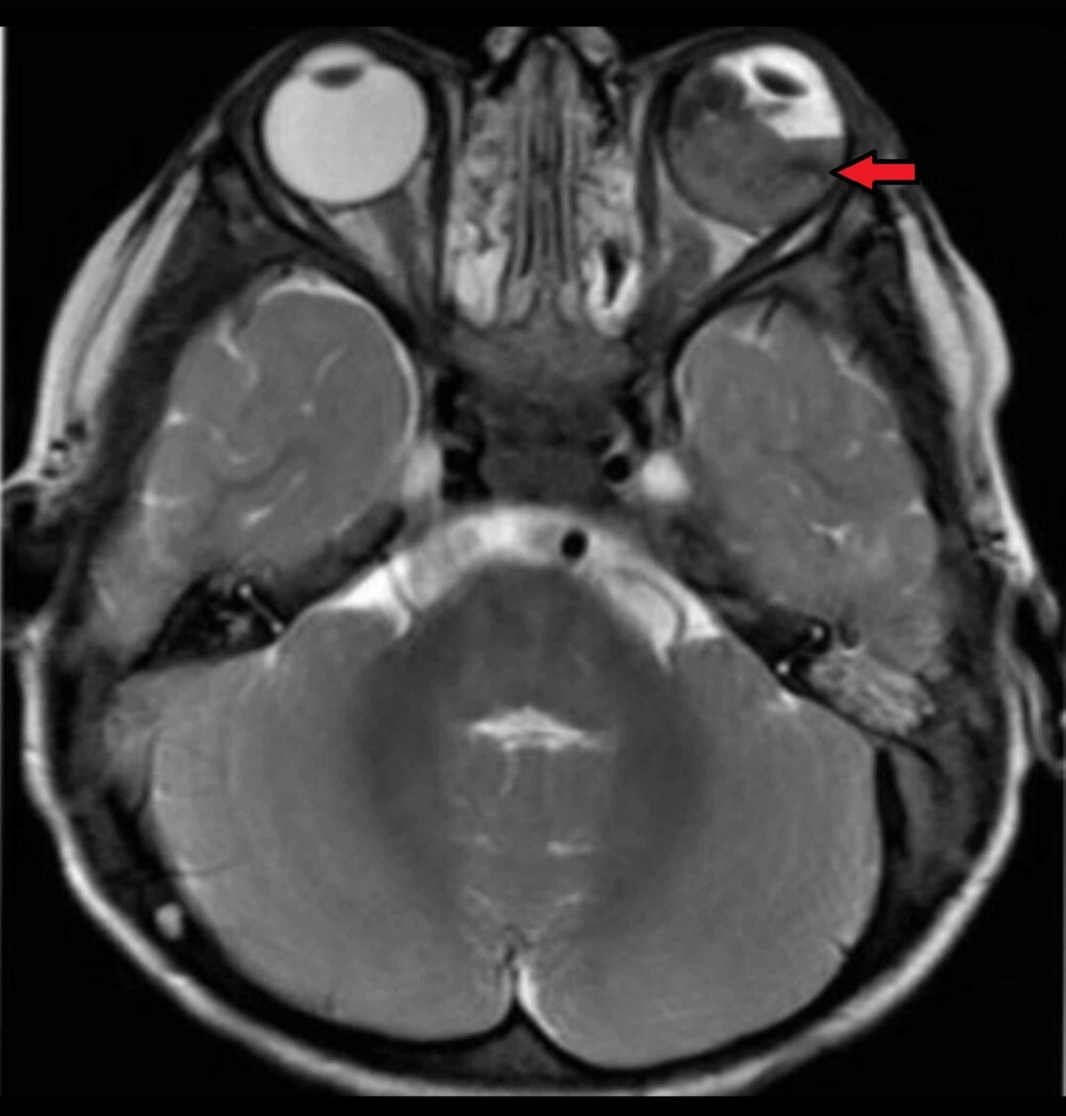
Axial T2-weighted MRI of the brain and orbit showing retinoblastoma in left eye along with retinal detachment (red arrow)
